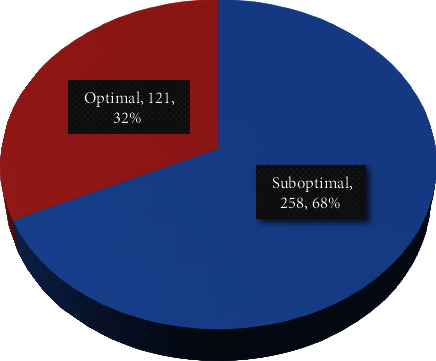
Contrast enhancement adequacy for patients with postcontrast abdominal CT at TASH with optimal defined as >50 HU increase from precontrast to portal venous phase, 2022 G.C.
